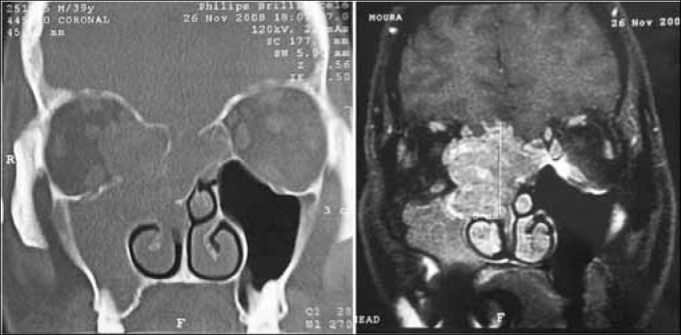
Preoperative CT and MRI of patient IGM, stage Kadish C and Dulguerov T3n0.
